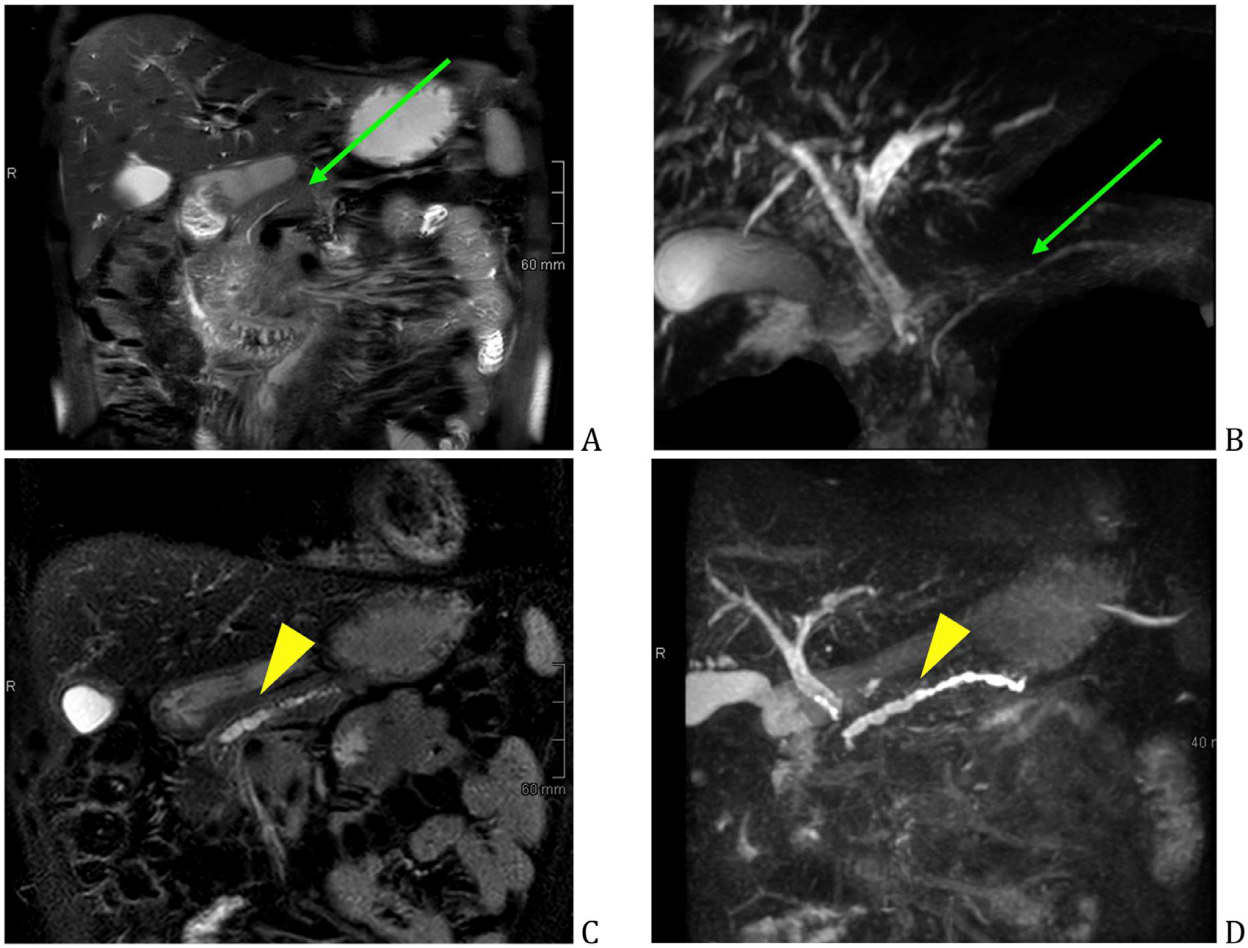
Follow-up MRI/MRCP - span of 2 months, main pancreatic duct dilatation. Initial work-up – no main pancreatic duct dilatation (green arrow) (A, B), follow-up after 2 months—apparent main pancreatic duct dilatation (yellow arrowhead) (C, D).
